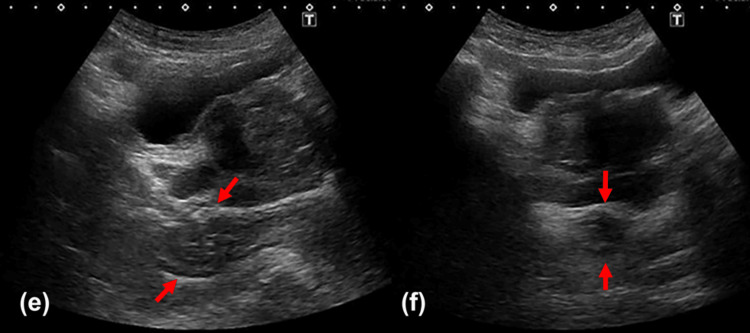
Short-axis and long-axis ultrasonographic images of the rectum on day 5 (e) In the long-axis view, the posterior wall of the rectum was visualized. Due to bowel collapse, the layered structure of the rectal wall was prominent (indicated by the red arrow). (f) In the short-axis view, the posterior wall of the rectum was also visualized (indicated by the red arrow). Compared to day 1, the rectal distension measured approximately 22 mm, indicating a decrease in luminal diameter. The patient was examined in the supine position. Images were obtained using an Aplio 500 ultrasound system (Canon Medical Systems Corporation, Otawara, Japan) with a convex probe set at 3.5 MHz
